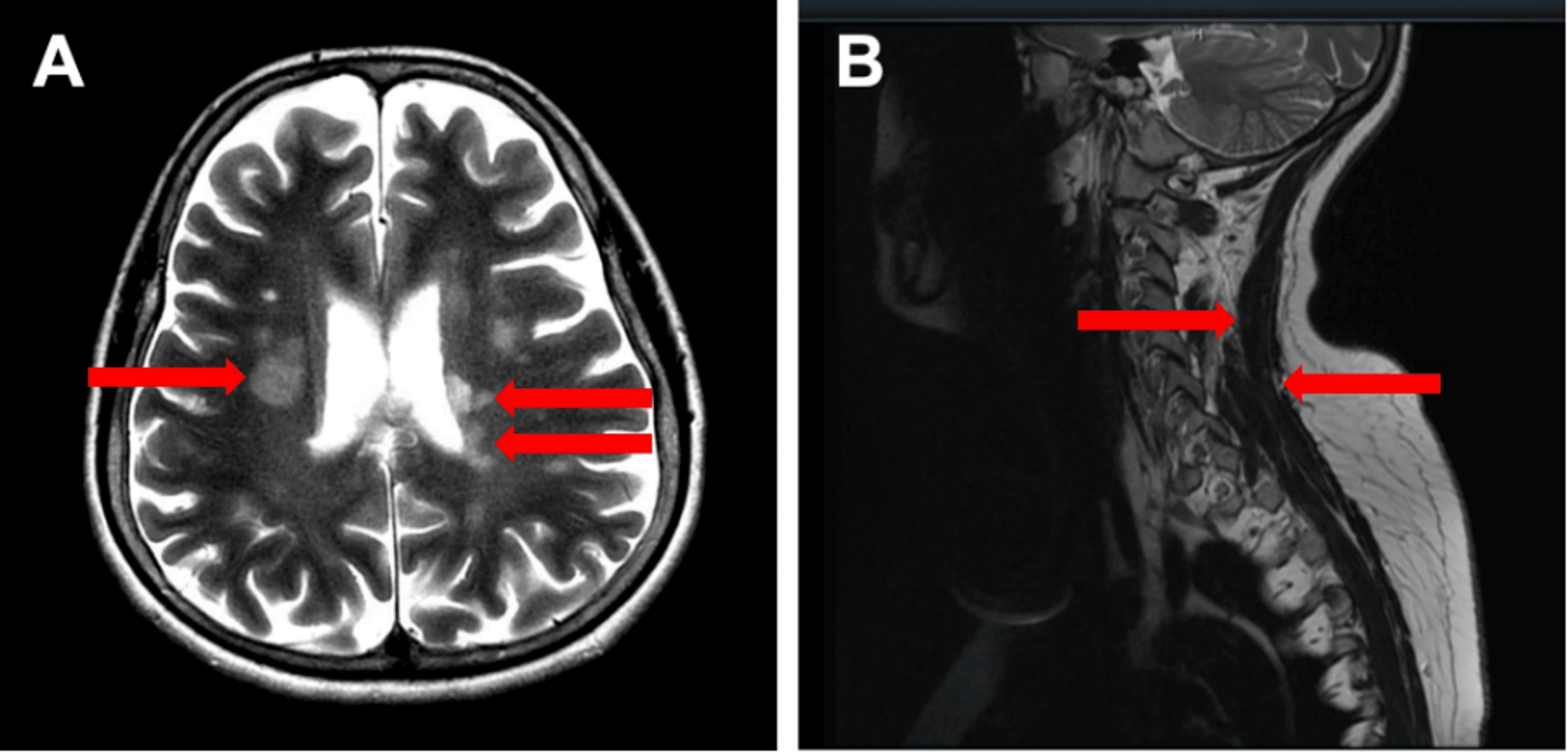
(A) T2-weighted axial MRI of the brain shows hyperintense periventricular lesions (red arrows). (B) T1-weighted sagittal MRI of the cervical and thoracic spine shows hyperintense lesions (red arrows)
